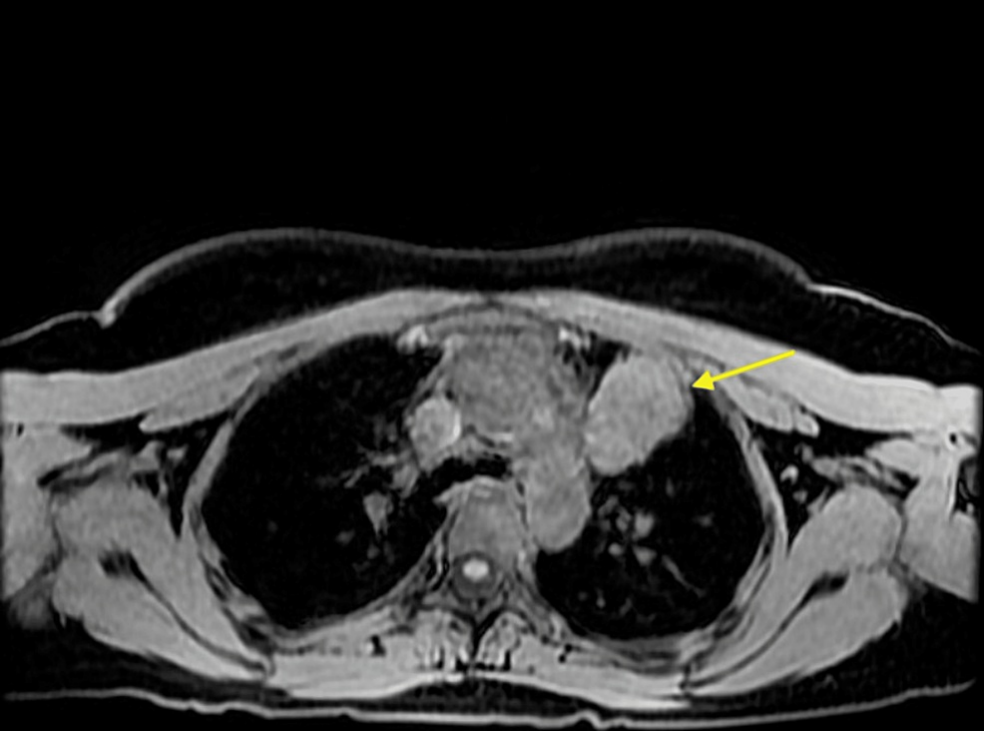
MRI thorax axial view showing a thymoma. MRI: magnetic resonance imaging.
